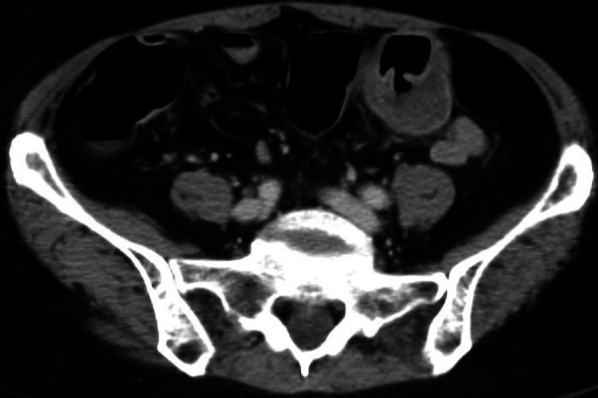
Contrast-enhanced computed tomography revealed a soft tissue with a poor central contrast effect localized to the sigmoid colon. The tumor had a depression suspected to be an ulcer. There was no evidence of surrounding tissue infiltration, free air, or ascites
